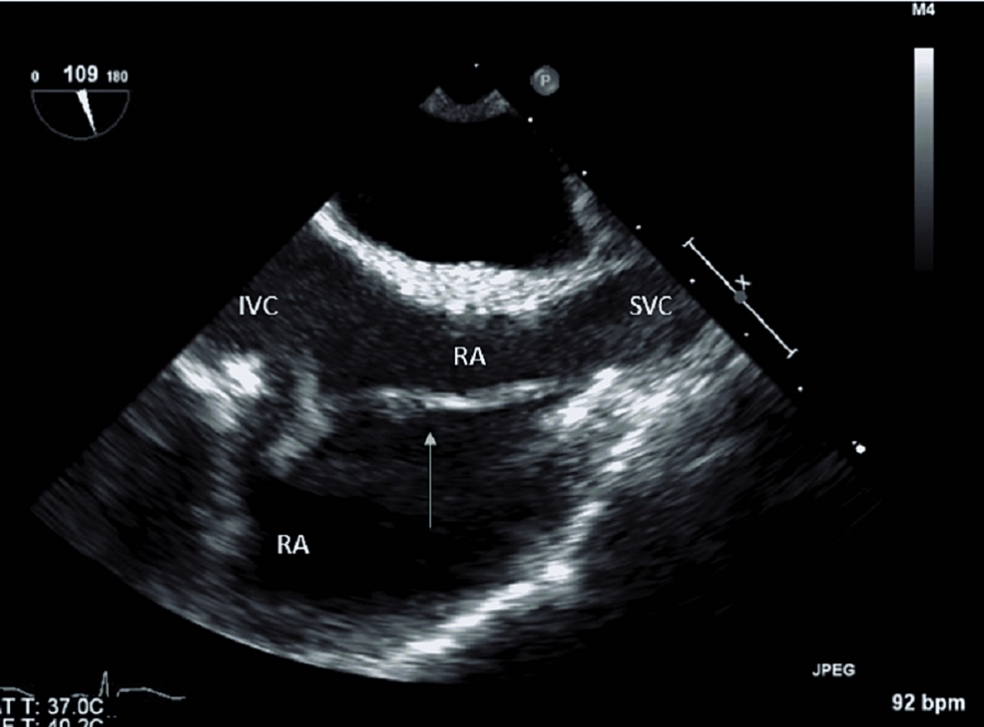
Initial trans-esophageal echocardiogram (TEE) showing the biofilm extending along the right atrium and associated with a peduncle. RA, right atrium; IVC, inferior vena cava; SVC, superior vena cava
